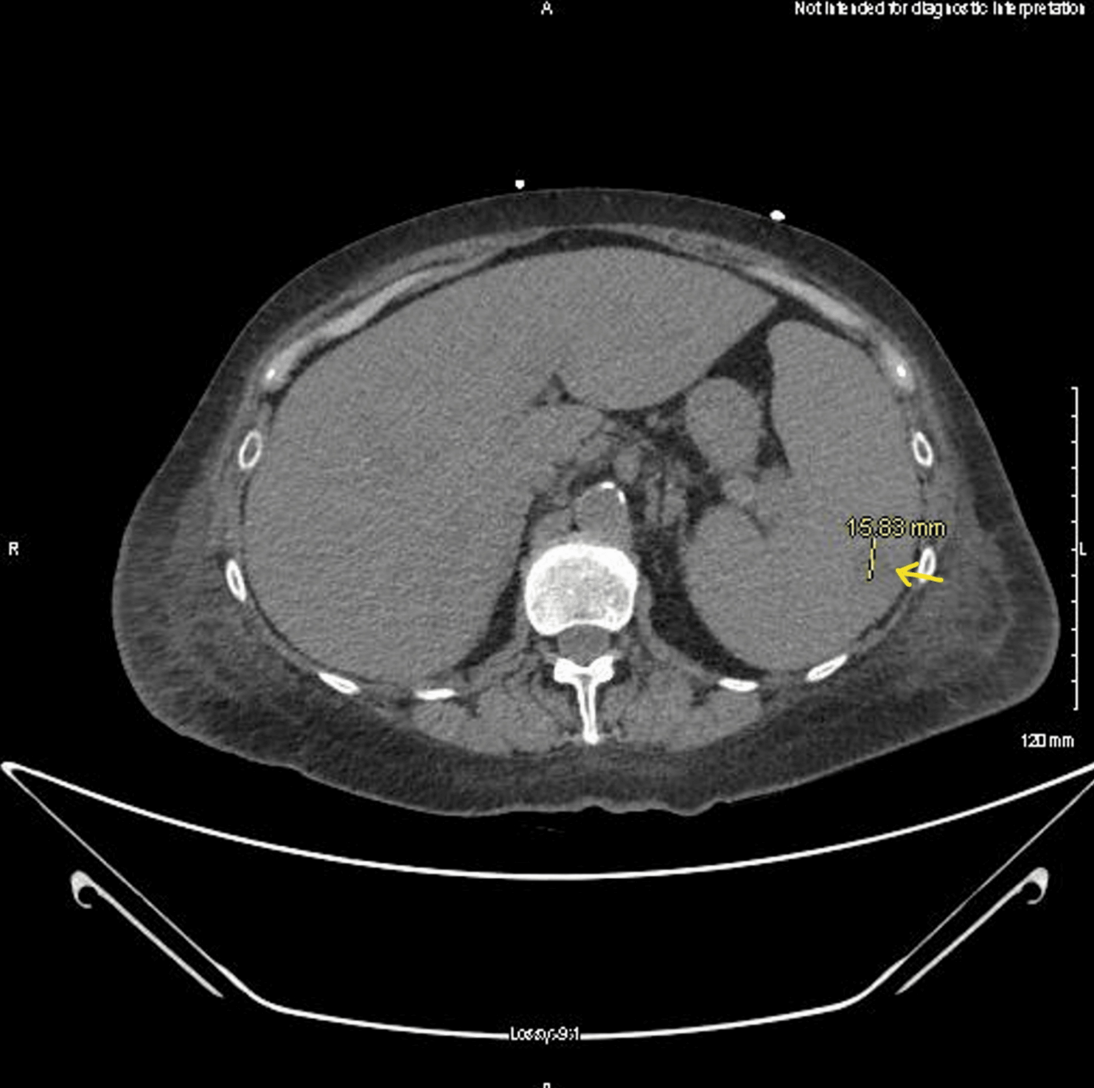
CT of the abdomen and pelvis: splenic hypoattenuating lesion with splenomegaly
